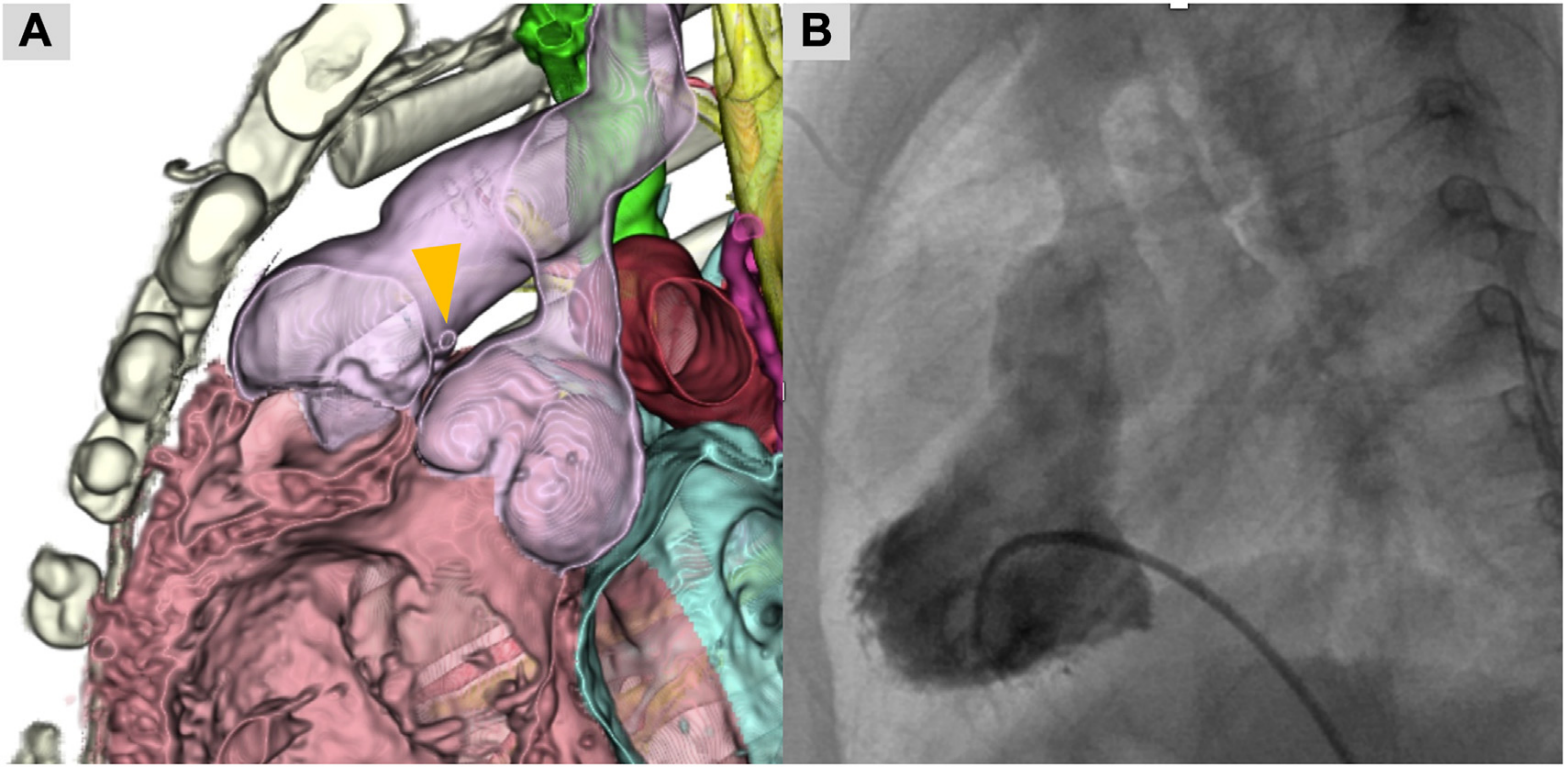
Postoperative computed tomography (CT) and catheter examination. (A) CT shows no stenosis at the Damus-Kaye-Stansel (DKS) anastomosis site (arrowhead) or coronary artery obstruction. (B) A postoperative catheter examination shows a smooth DKS pathway.
